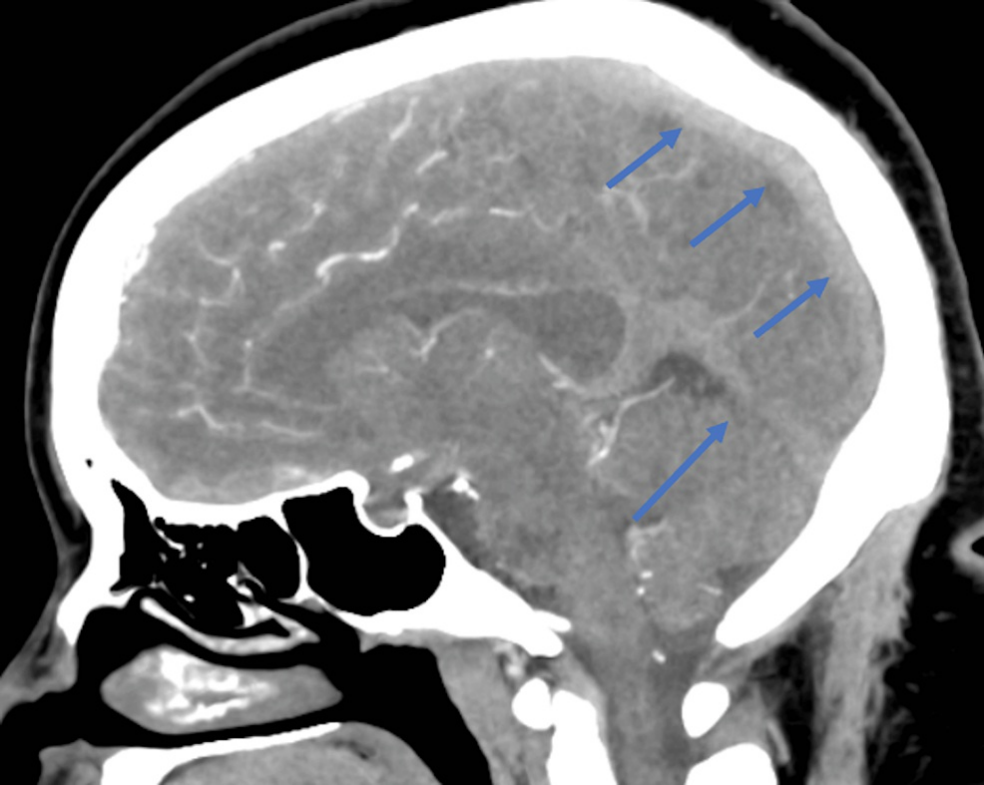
CT angiogram of the head and neck There is evidence of extensive dural sinus and deep venous thrombosis (blue arrows), although the study is not optimized to evaluate venous structures. There is a lack of evidence of arterial stenosis, aneurysm, or arterial venous malformation CT: computed tomography
